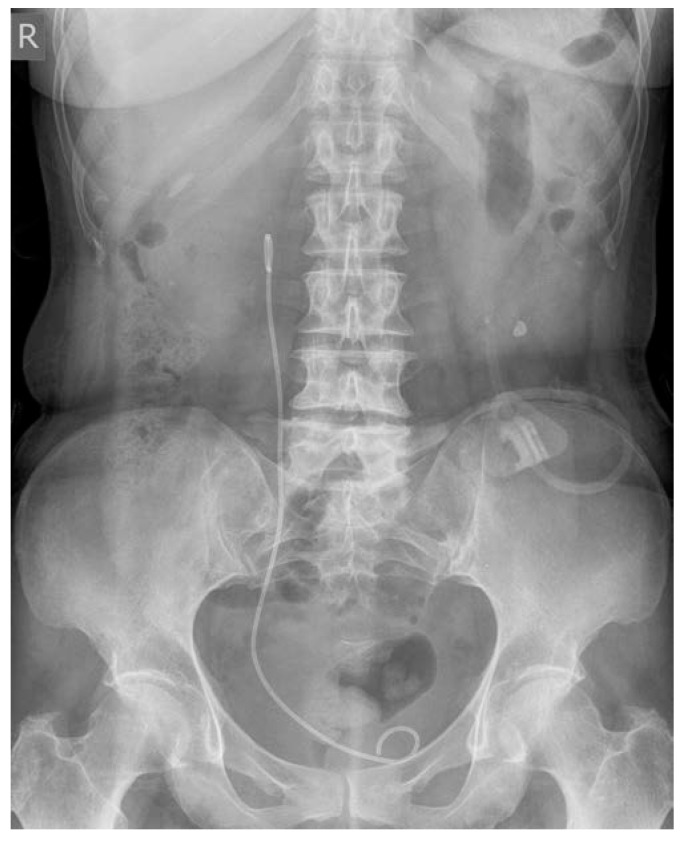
Abdominal X-ray revealing the correct position of the Cook catheter.
